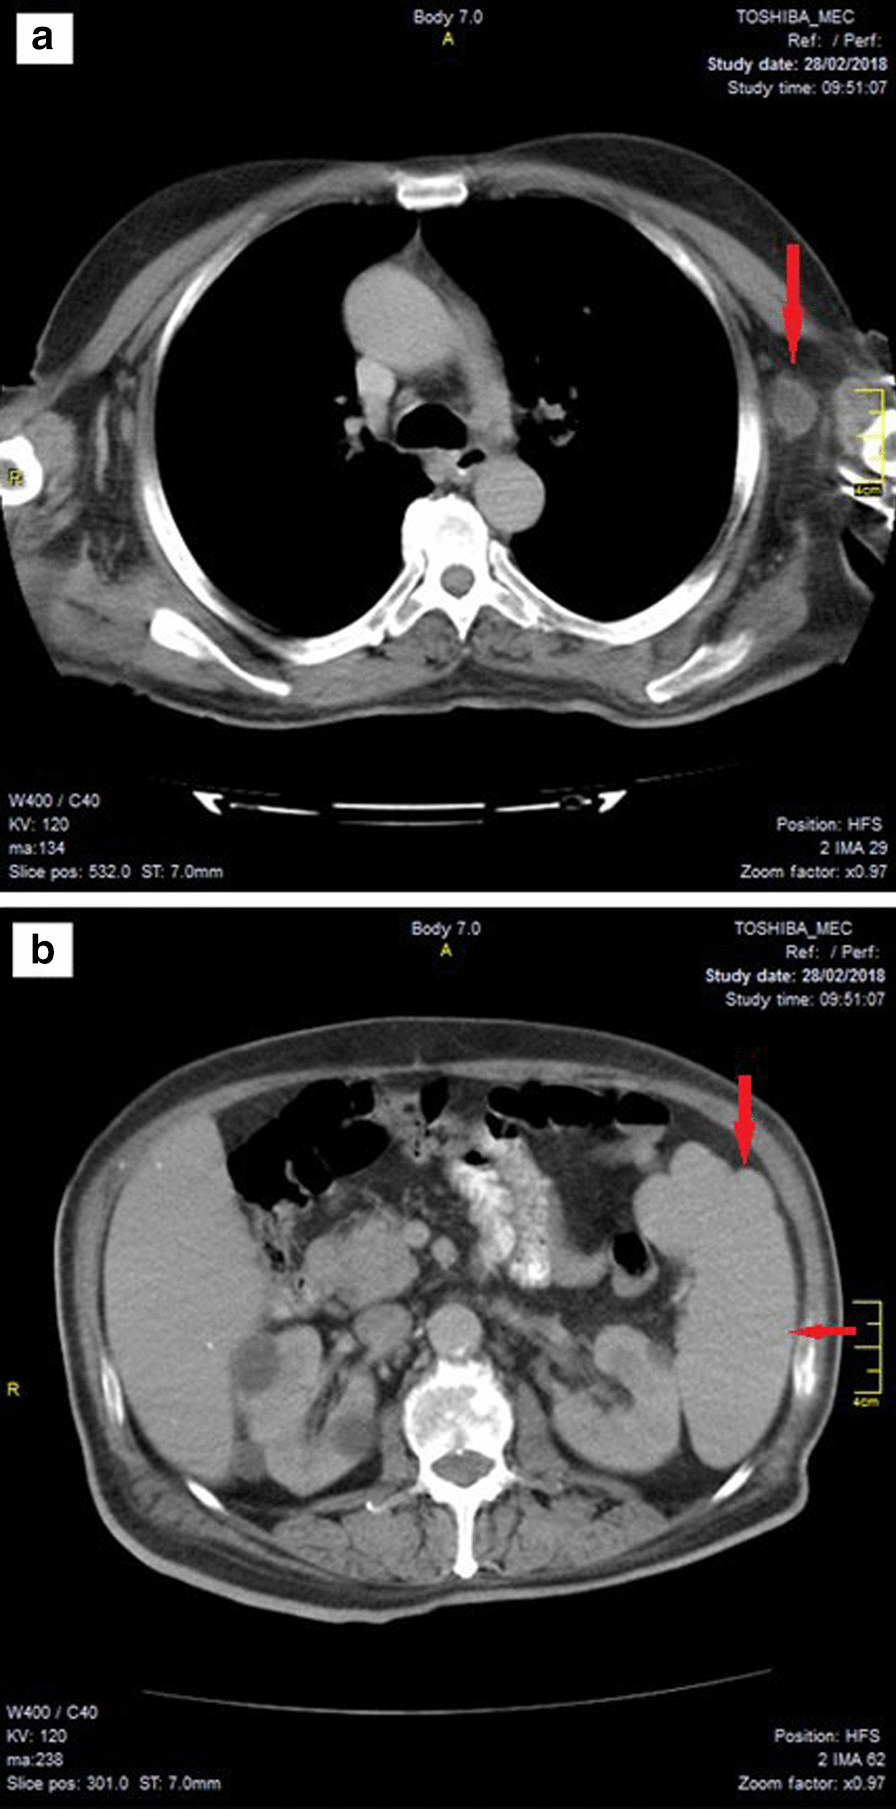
Computed tomography scan with IV contrast of the thorax and abdomen acquired 2 months after the initiation of treatment, showing left axillary nodule measuring 3.5 cm in diameter [a—arrow] and a mild splenic enlargement [b—arrows]
